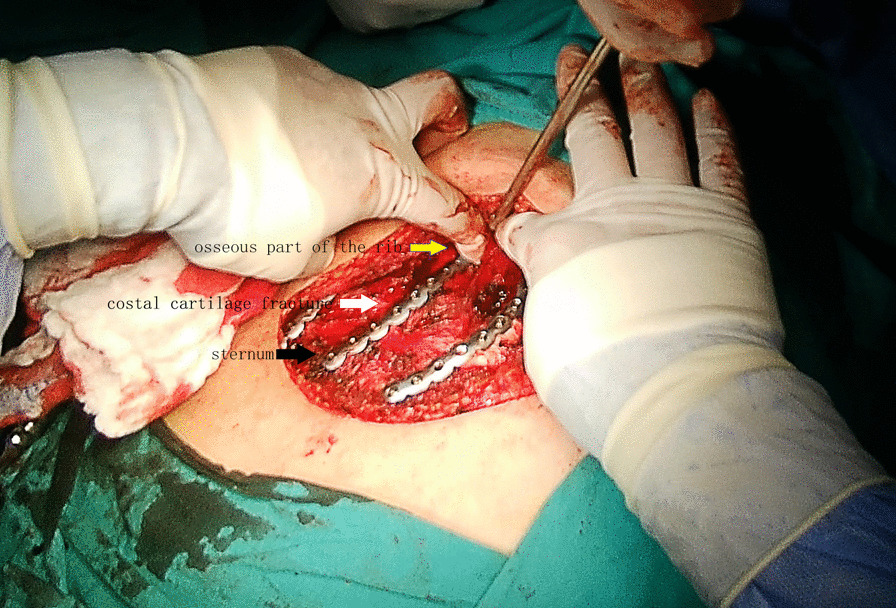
CT Showing a cured costal cartilage fracture 6 months after operation
